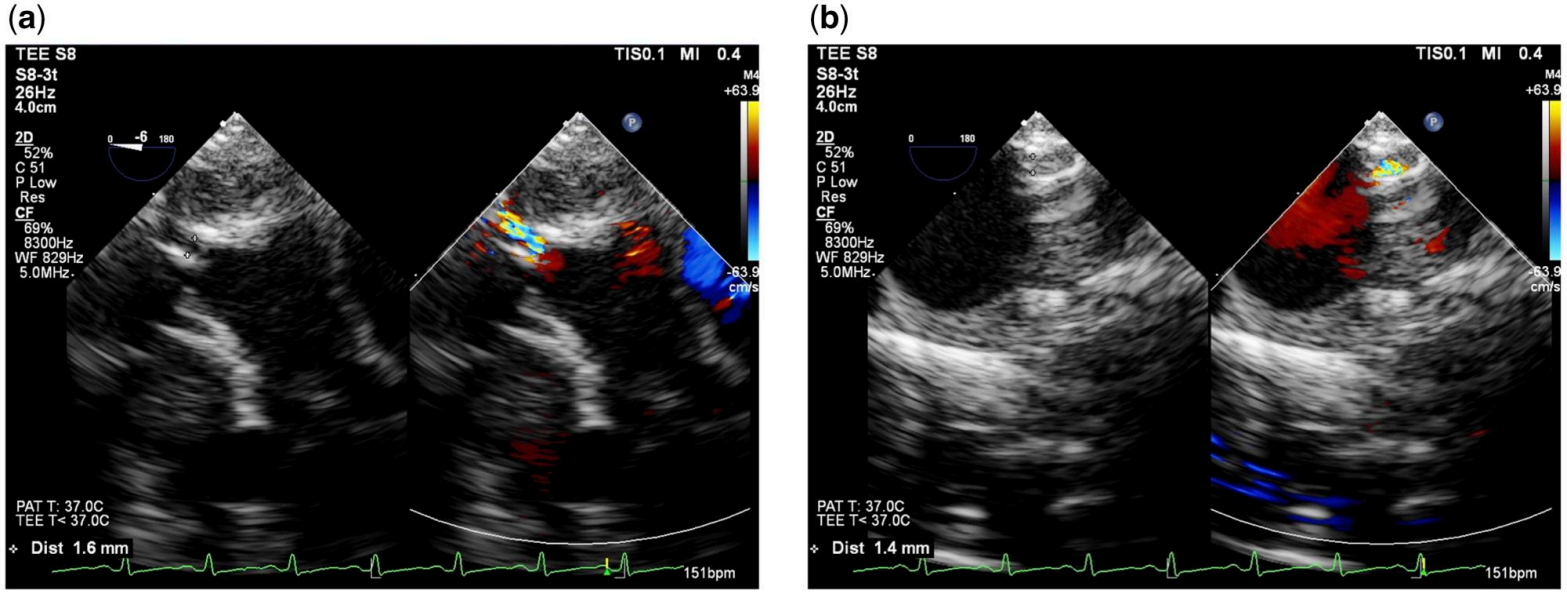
Adjustment of the bilateral pulmonary artery using transoesophageal echocardiography. The target lumen diameter was about 1.5 mm. (a) Right pulmonary artery after banding. (b) Left pulmonary artery after banding.
